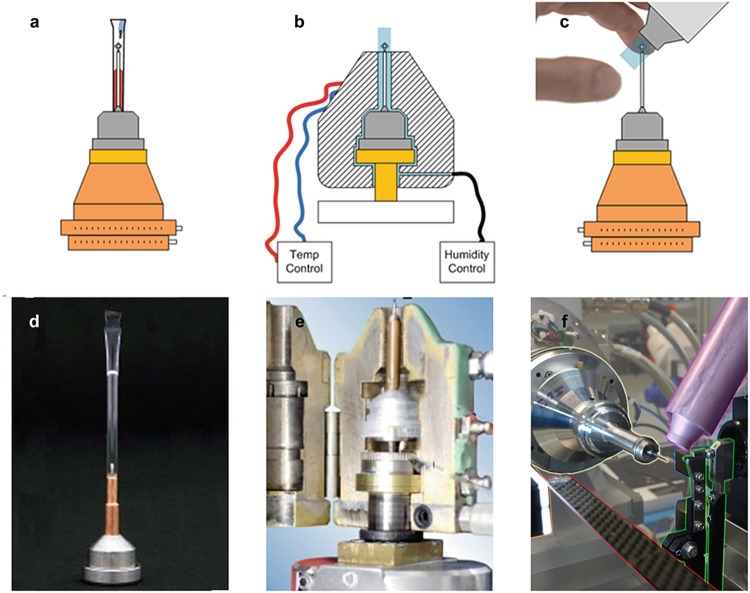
Methods of dehydration with X-ray feedback. (a) and (d) Capillary dehydration. (b) and (e) Free Mounting System. (c) and (f) The humidity control dehydration device (HC1)
